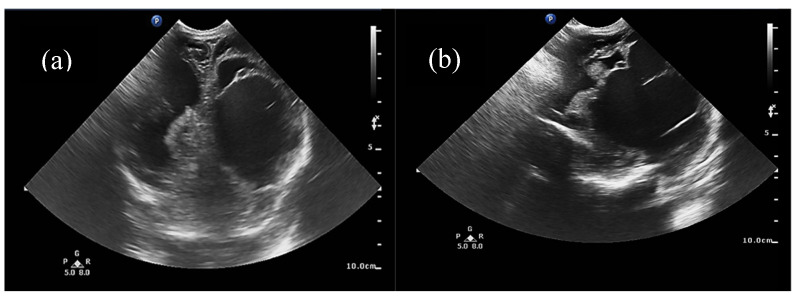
(a) Two-dimensional ultrasound of the VoGM via anterior fontanelle, (b) 2D ultrasound of the VoGM via the mastoid fontanelle. Voluminous, multilobulated, transonic mass of 6/3 cm, exerting a compressive effect on the left lateral ventricle, with slight displacement of the median structures.
